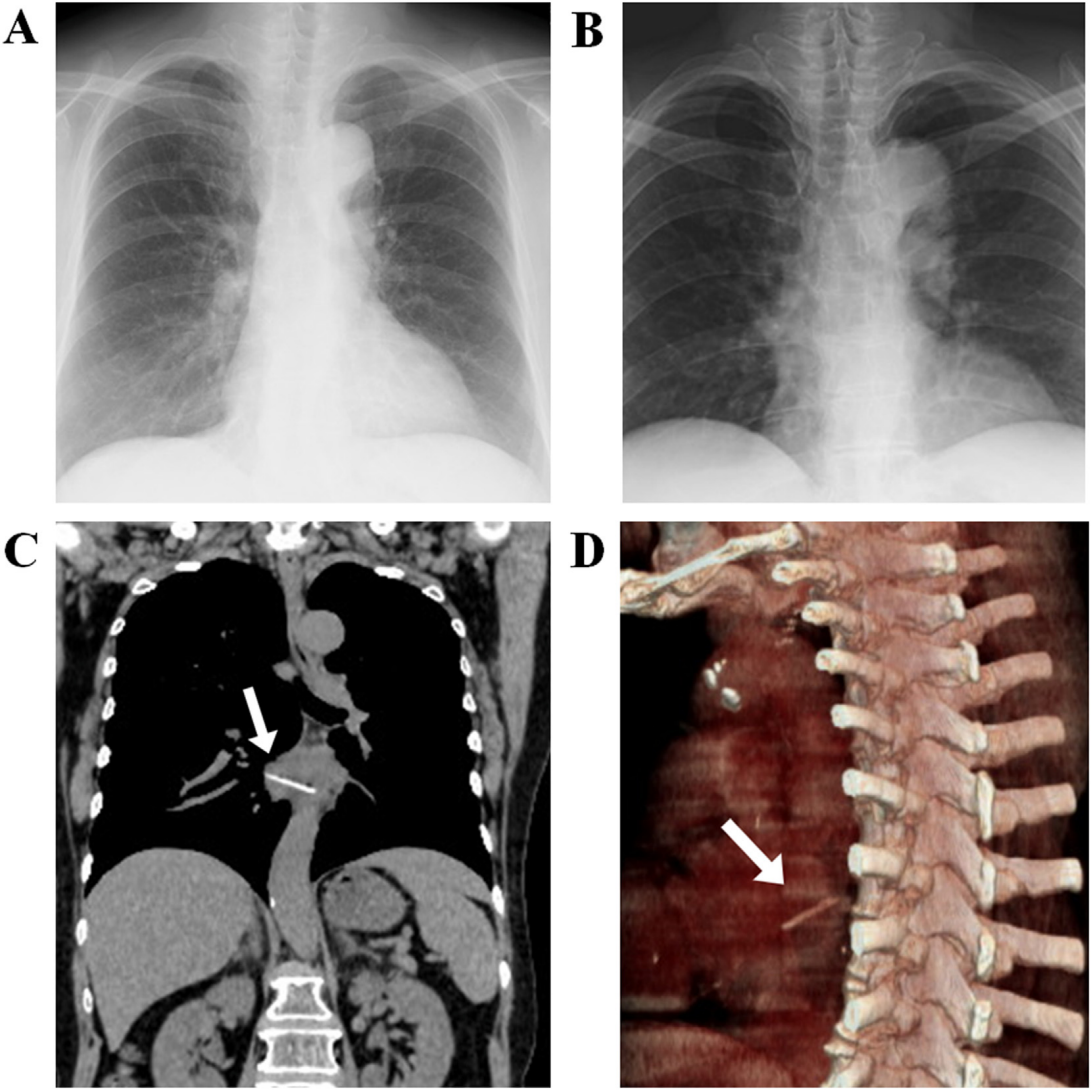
Chest X-ray and non-contrast chest computed tomography. (A, B) Chest X-ray failed to detect any abnormal findings, including the supposedly lodged fish bone in the esophagus. (C, D) Non-contrasted chest CT revealed a large fish bone impacted in the middle esophagus, penetrating the esophageal wall into the surrounding mediastinal structures.
